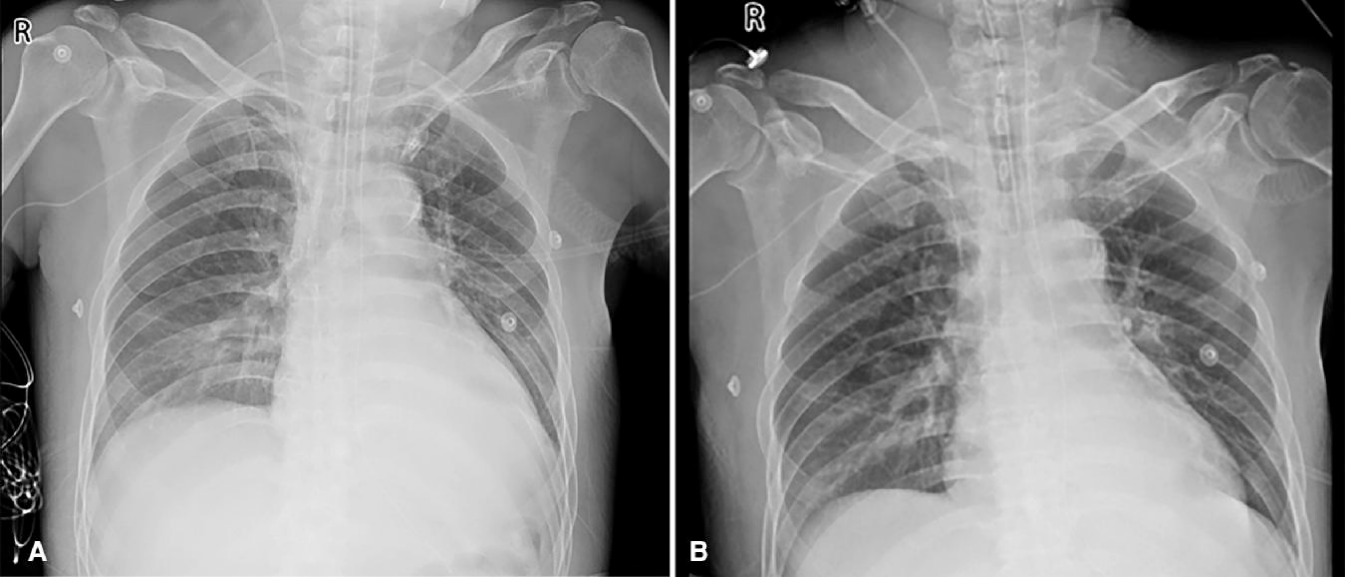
Fig.3A is the chest X-ray of the patient upon admission to the ICU, and Fig.3B is the chest X-ray before the patient's discharge. The images reveal increased lung markings, a small amount of pleural effusion on both sides and patchy blurred shadows in the right lower lung field.
